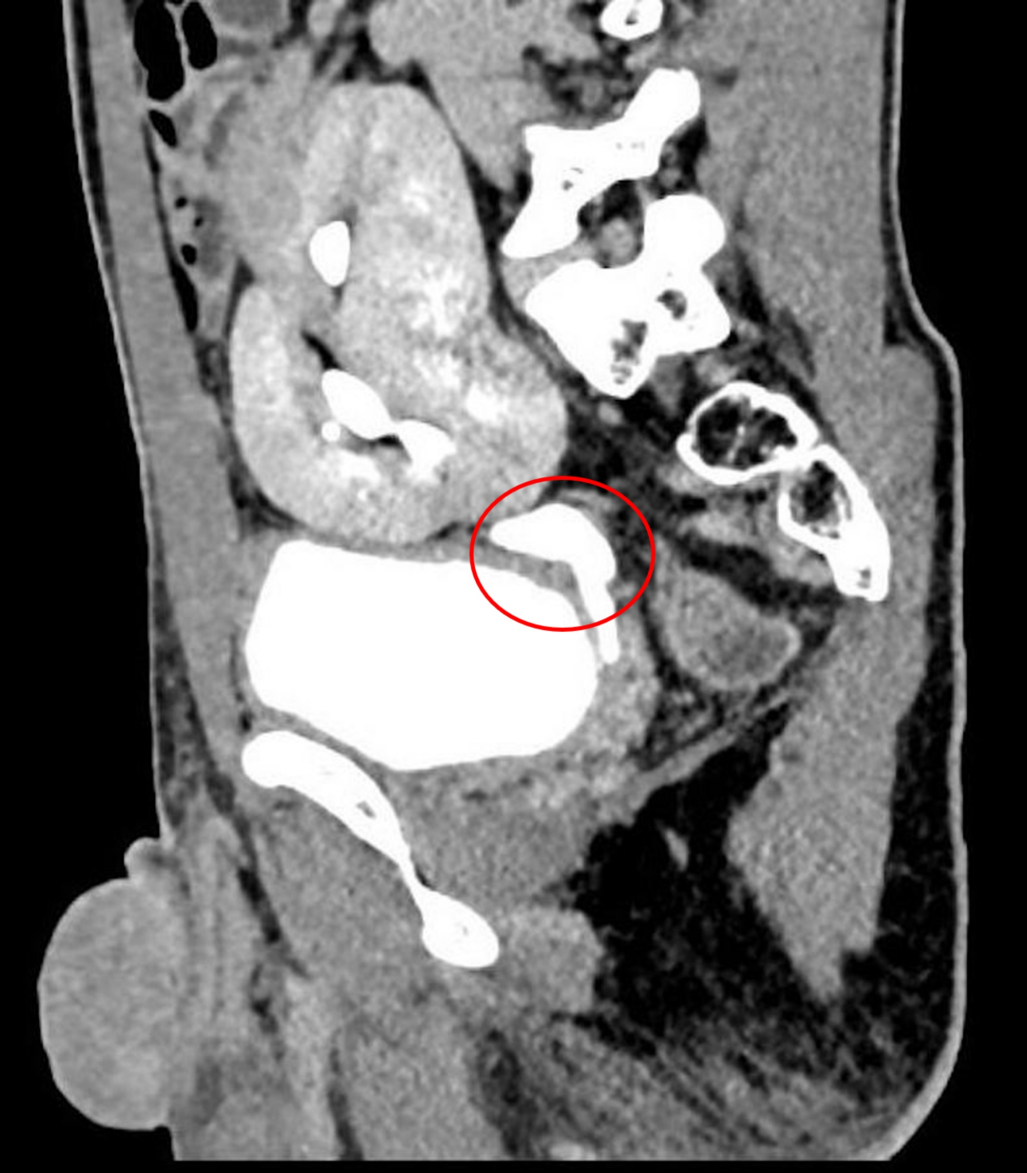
Computed tomography revealing a single ureter draining the collecting system of both the kidneys and terminally opening ipsilaterally into the urinary bladder.
